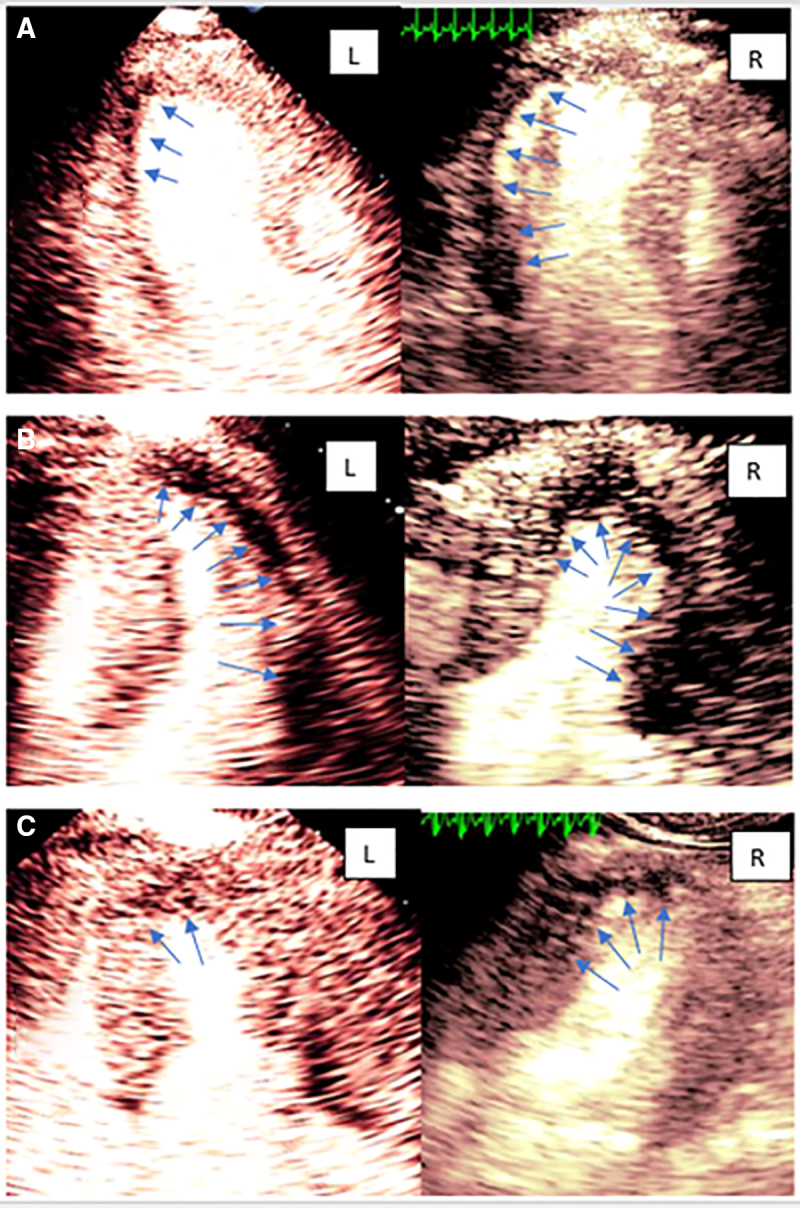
Conventional myocardial contrast echocardiography (MCE; left) and high frame rate (HFR) MCE (right) end-systolic images. A, Occluded single obtuse marginal artery (OM) artery. Three-chamber view: the arrows demonstrate perfusion defect within inferolateral wall. HFR image shows more extensive and intense perfusion defect with underlying wall thickening abnormality compared with conventional MCE in a patient with single vessel occluded large OM. B, Four-chamber view. HFR demonstrates moderate intensity perfusion defect of mid to distal inferoseptal and apex and severe defect of the anterolateral wall. Corresponding conventional MCE shows less extensive apical defect with a large anterolateral defect. The patient had mid left anterior descending artery (LAD) and a large caliber left circumflex artery (LCx) obstructive coronary artery disease. C, Four-chamber view MCE of a patient with severe, single vessel, proximal LAD artery stenosis. HFR image demonstrates more extensive and intense perfusion defect within mid to apical infero-septal wall compared with conventional MCE. L indicates left; and R, right.
